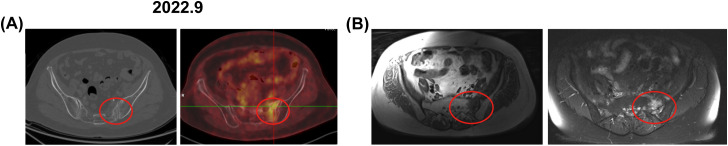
Bone changes observed in the patient from case 2. September 2022: (A) Enhanced CT and PET-CT images revealed increased metabolic activity in the left sacrum, with a SUV of 4.6. (B) An axial T1-weighted MRI image displayed low signal intensity in the left sacrum, axial and coronal fat-suppressed T2-weighted images exhibited patchy long T2 signals. The red circle marks the area of bone change. CT, computed tomography; PET-CT, positron emission tomography-computed tomography; MRI, magnetic resonance imaging.
